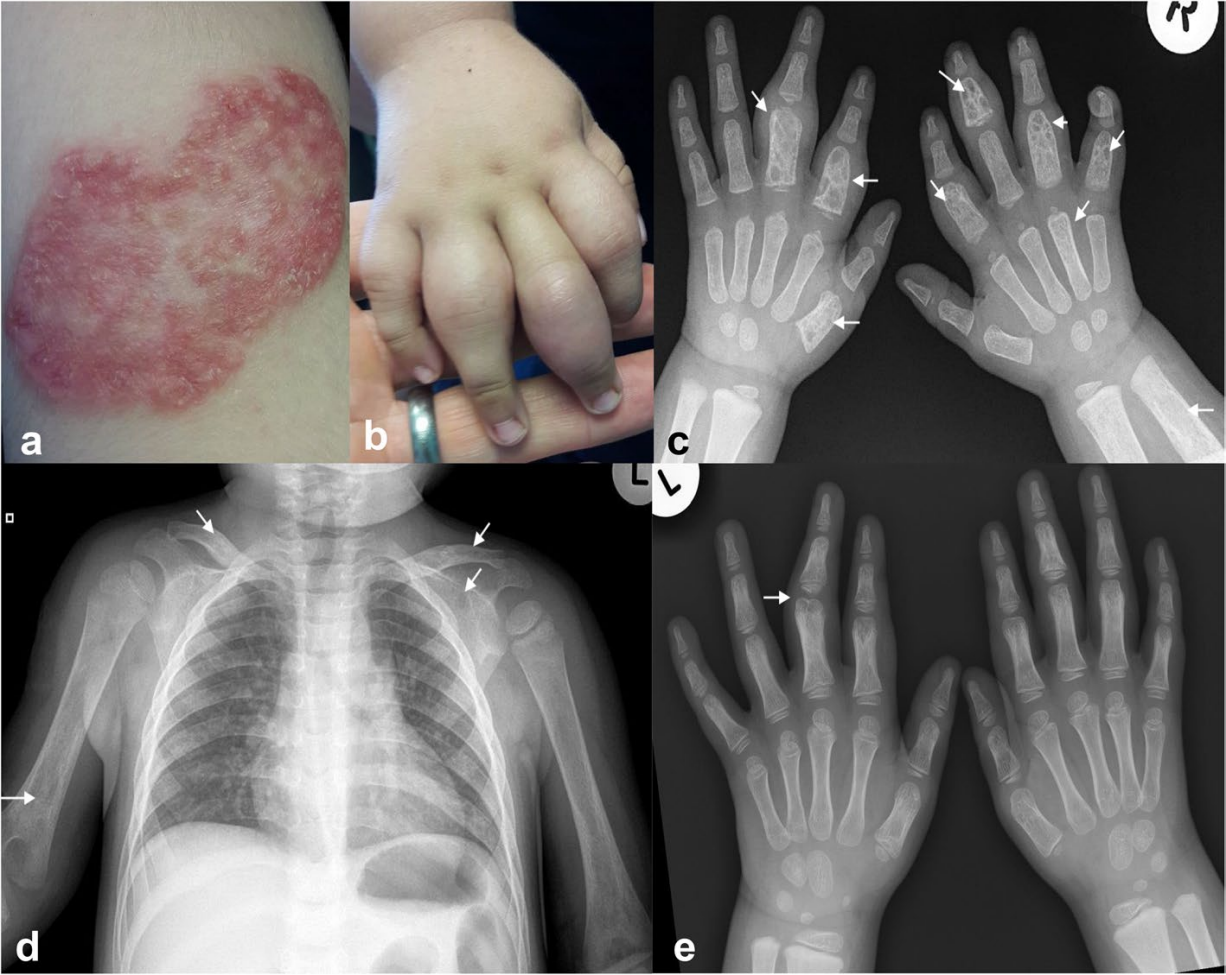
Clinical and radiological features of the proband. a Confluent non-healing plaque-like lesion, over the right deltoid muscle at the site of BCG vaccination, from which *Mycobacterium bovis* BCG was cultured. b Right hand, showing non-erythematous swelling over the 2nd, 3rd, 4th and 5th proximal interphalangeal joints. c AP radiograph of both hands demonstrates multifocal osteomyelitis with bone expansion and multiple ill- and well-defined lucencies of varying sizes affecting the left 1st metacarpal, left 2nd and 3rd proximal phalanges, right 2nd, 3rd, 4th and 5th proximal and middle phalanges with associated soft tissue swelling. The right 4th metacarpal and distal right radius and ulna shows bone expansion and small ill-defined lucencies. d Antero-posterior (AP) chest radiograph demonstrates multifocal osteomyelitis with bone expansion and ill-defined lucencies in the right distal humerus, both clavicles and left scapula. The heart and lungs are normal and there is no intrathoracic lymphadenopathy. e AP radiograph of both hands 2 years after presentation demonstrates interval resolution of the features of multifocal osteomyelitis. Healing deformity of left 3rd finger
